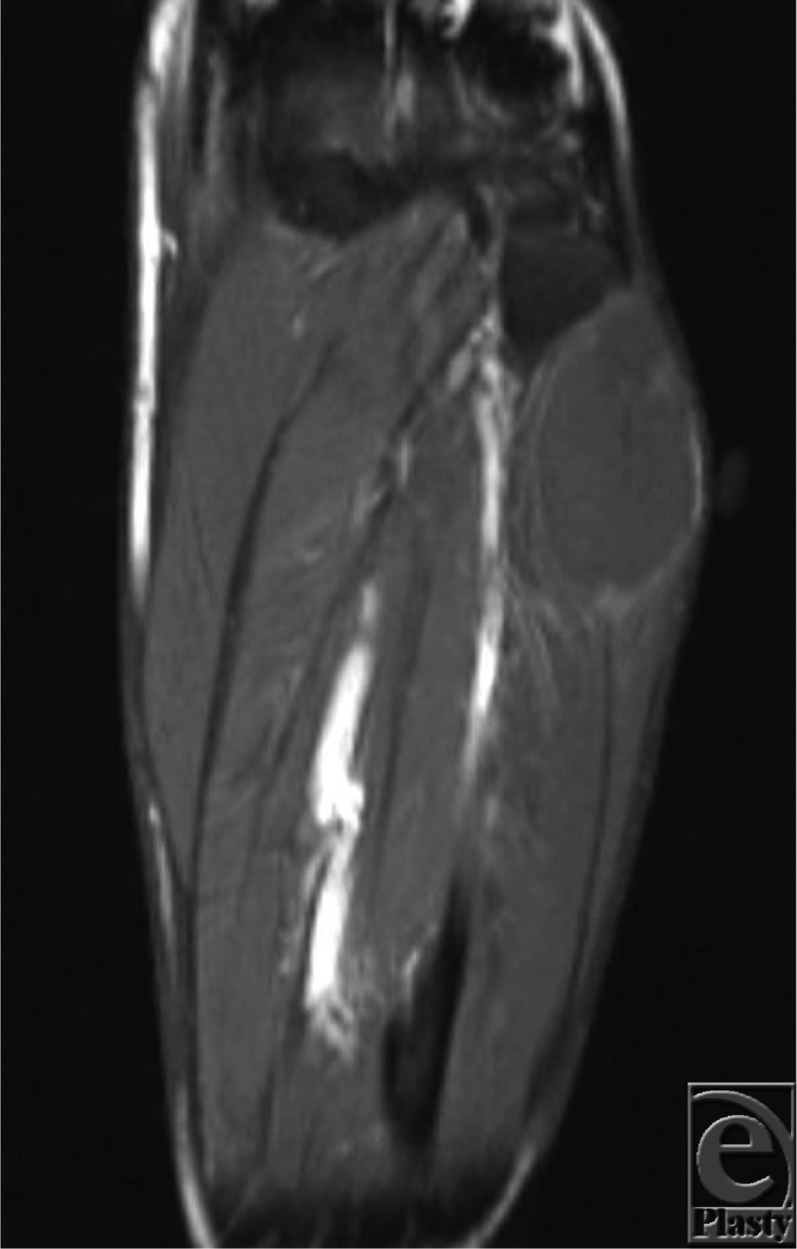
Coronal contrast-enhanced, T1-weighted magnetic resonance image of the peroneus longus ganglion cyst.
